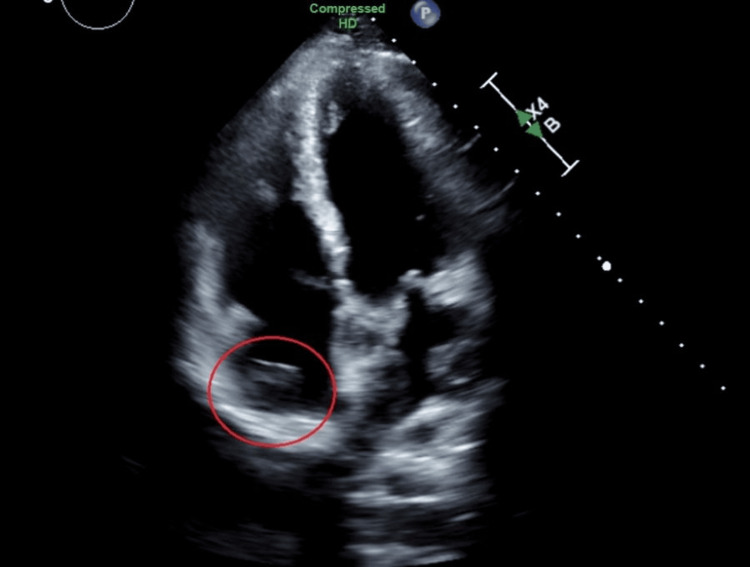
Transthoracic echocardiogram (TTE), apical four-chamber view, demonstrating an echogenic right atrial mass (circled).
